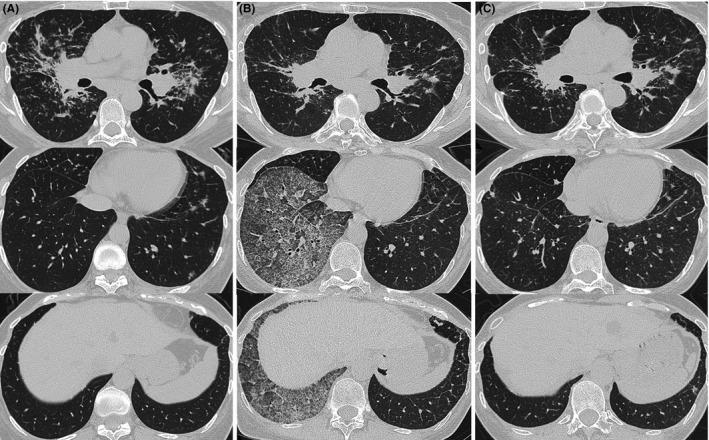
High‐resolution computed tomography images on the first visit showed bilateral multiple perilymphatic nodules (A). Eight years later, a crazy‐paving appearance was seen on the right lower lobe (B). Four months later, after video‐assisted thoracic surgery (VATS), the crazy‐paving appearance was markedly improved (C)
